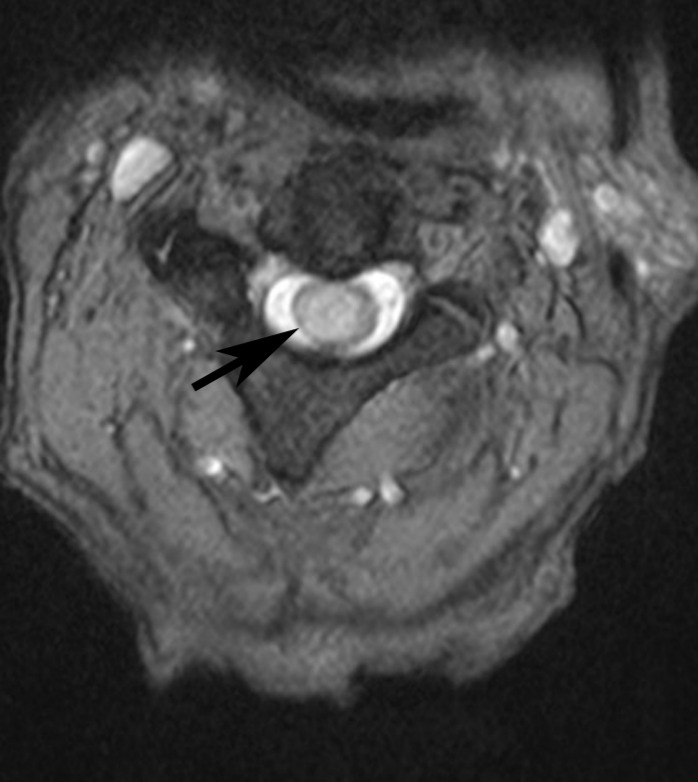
Axial T2-weighted MRI of his cervical spine showed that the lesion is slightly to the right of the midline.
